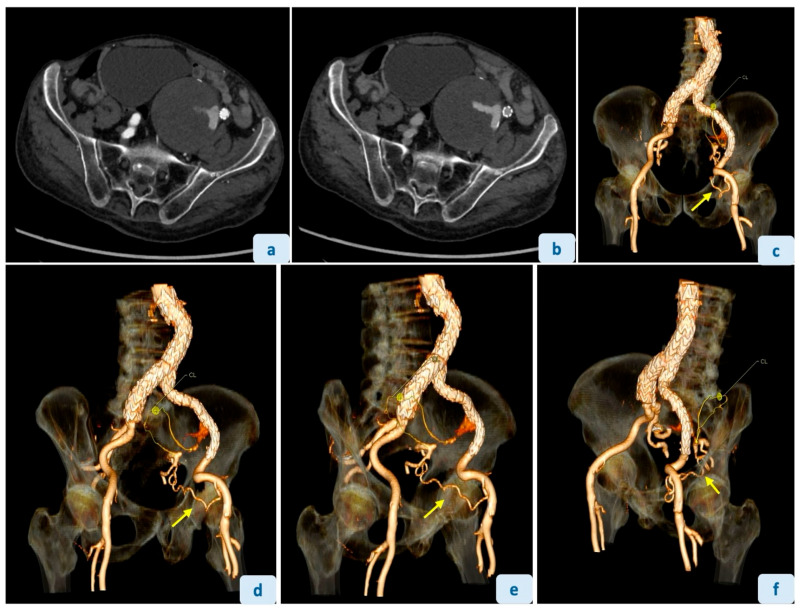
An 88-year-old male with a history of open aorto-aortic graft for ruptured abdominal aortic aneurysm (2021) and subsequent development of a left CIAA treated with EVAR (2023), presented to our Emergency department with diffuse abdominal pain and vomiting, raising suspicion of intestinal subocclusion. Computed tomography angiography (CTA) confirmed a large left-sided CIAA, increased in size compared with 2023 imaging (84 vs. 61 mm in axial diameter), without signs of rupture or fissuring, involving the entire length of the CIA up to the origin of the IIA. The increase in size was attributed to the presence of an EL2 originating from the ipsilateral iliolumbar artery (a,b) with a tortuous course (yellow thin line with “CL” mark) (c–f). A careful review of the CTA identified a “corona mortis” variant (yellow arrow), with reperfusion of the excluded IIA (c–f). The case was discussed in a multidisciplinary meeting, including vascular surgeons. Although the patient’s abdominal pain was not attributable to the CIAA, the indication for treatment was based on significant aneurysmal sac enlargement (>2 cm in maximum diameter over approximately 2 years of follow-up), according to current European Society for Vascular Surgery (ESVS) guidelines [1]. The patient was considered not eligible for surgical repair due to age and comorbidities and was referred to our Interventional Radiology department for endovascular management. Given the prior exclusion of the IIA by the endograft, the “corona mortis” anastomosis provided a direct arterial pathway to access both the IIA and the iliolumbar branch supplying the aneurysmal sac.
